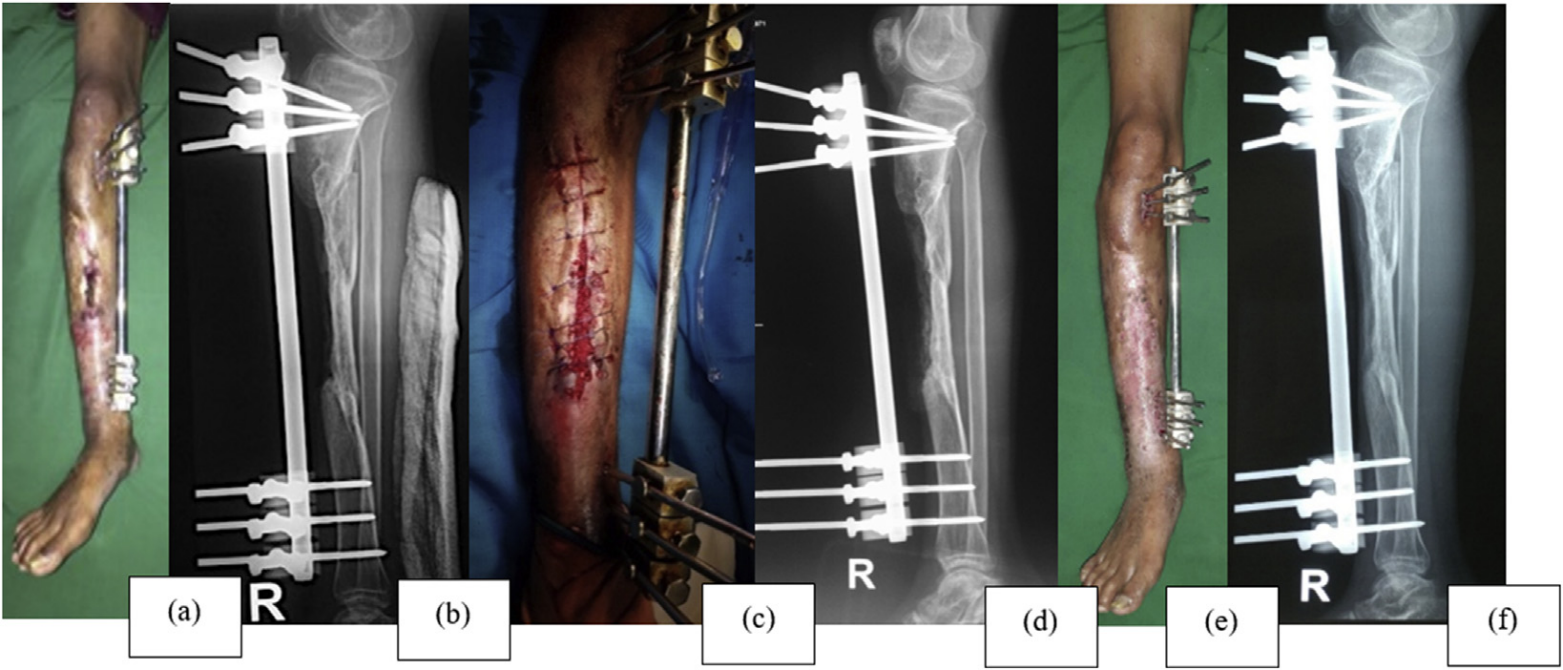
Case 4; a 27-year-old male with infected non-union of the right tibial shaft. (a) Anterior preoperative view of the right leg. (b) Preoperative lateral X-ray of the right leg. (c) Anterior view of the right leg. (d) Postoperative lateral X-ray of the right leg. (e) Anterior view of the right leg, 6 months postoperatively – healed wound. (f) Lateral X-ray of the right leg, 6 months postoperatively – bony union.
